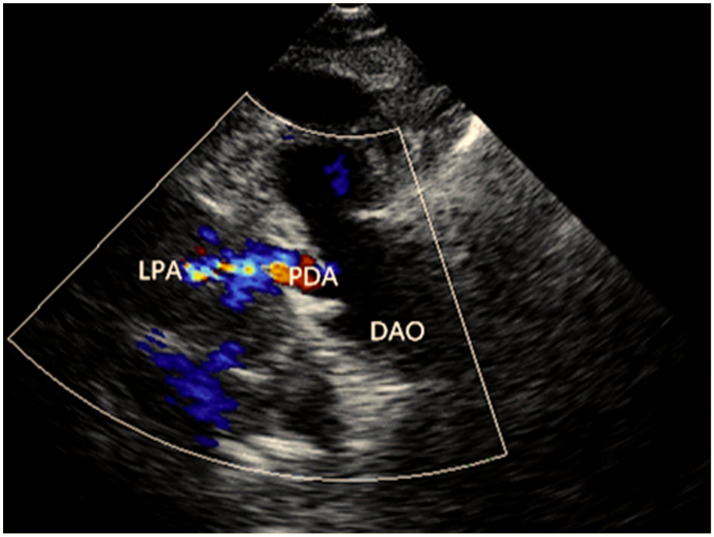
A representative echocardiographic image of the proband’s patent ductus arteriosus. The color flow image shows the presence of a patent ductus arteriosus between the left pulmonary artery and the descending aorta. DAO, descending aorta; LPA, left pulmonary artery; PDA, patent ductus arteriosus.
